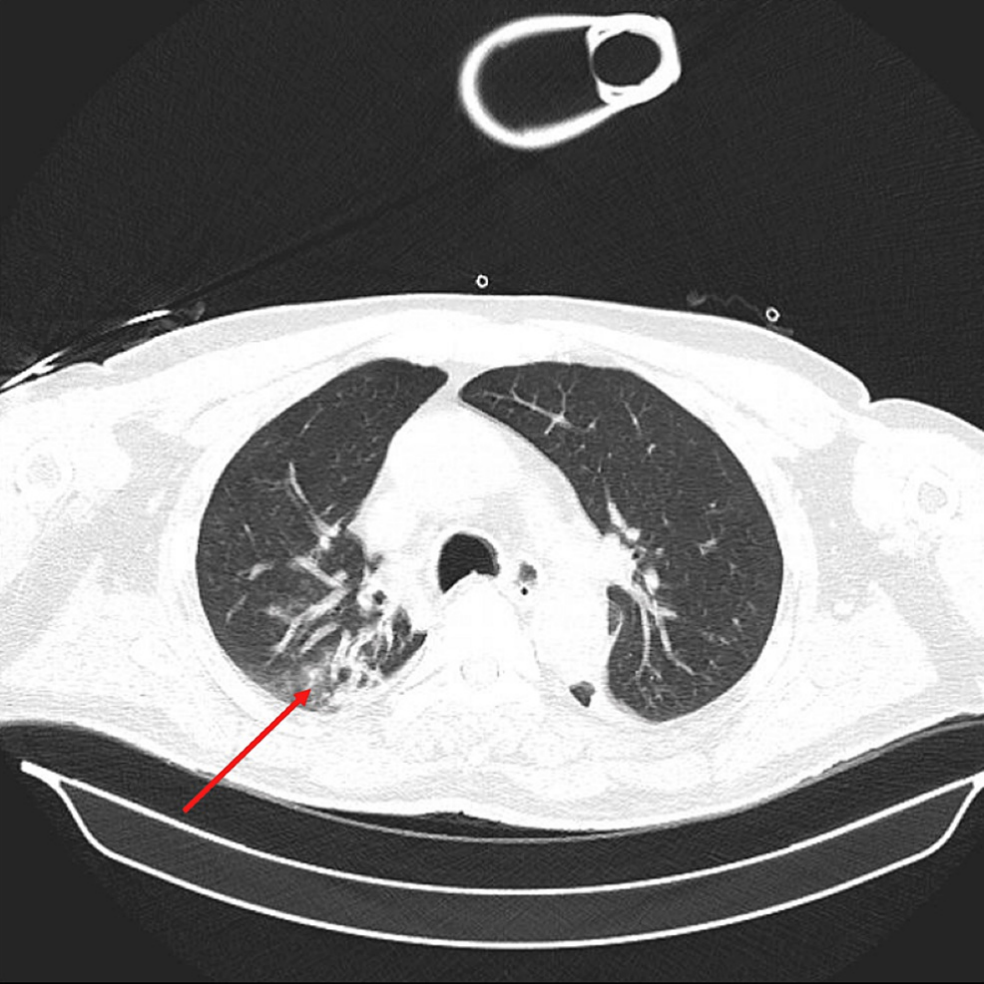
Pulmonary computed tomography of the patient.
